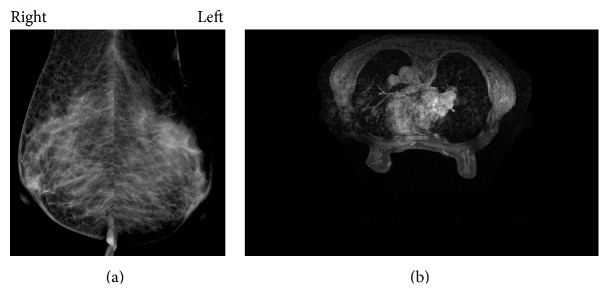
(a) Mammography. There was no lesion in either breast. (b) Enhanced MRI. There was also no lesion in either breast.
